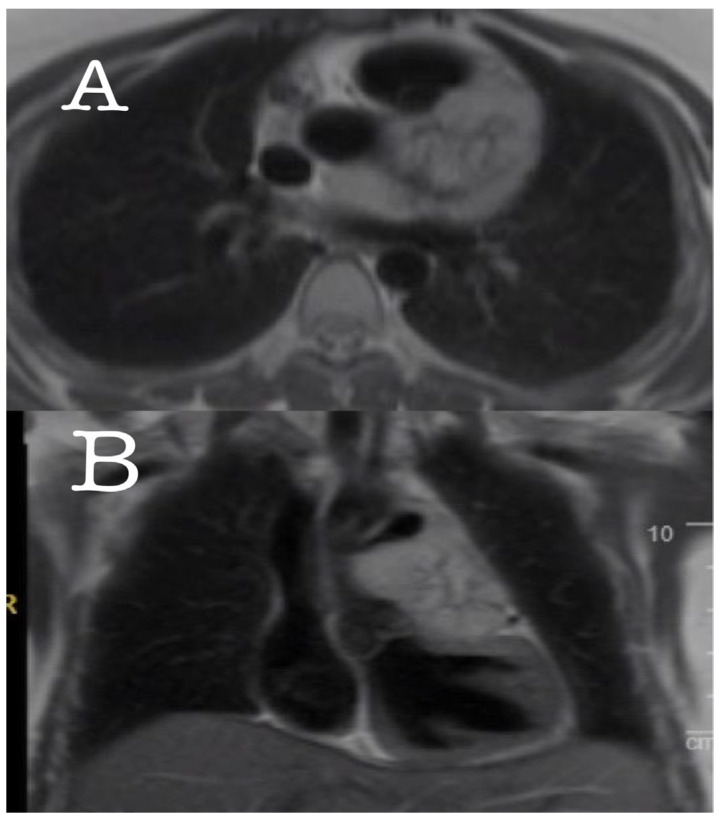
Axial (A) and coronal (B) MRI showed a mass encasing the LAD and the proximal LCX.
